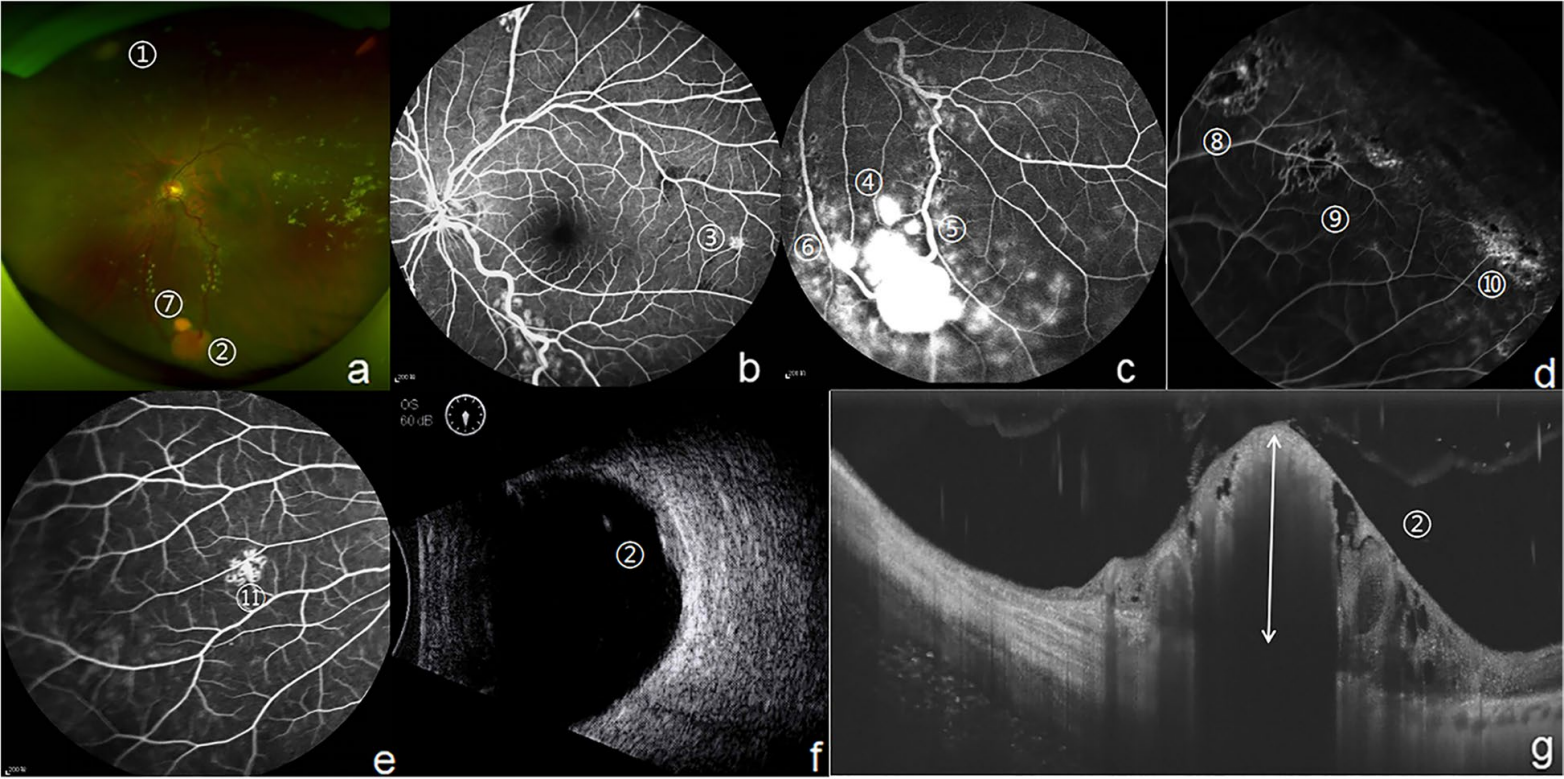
The first multimodal image evaluation of eleven RCHs in a Von Hippel-lindau syndrome patient on June 6^th^, 2017. a. Fundus photo identified huge RCHs (No.1, 2, 7 RCHs). b. FFA visualized a newly developed small RCH in temporal retina (No.3 RCH). c-e. FFA sketched the contours of several small peripheral RCHs (No.4–11 RCHs). f. B-scan ultrasonography displayed the largest oblate lesion with the size of 6 mm* 6 mm*2 mm was located in the inferior nasal retina (No.2 RCH). F. SS-OCT (single line scan mode) showed an elevated lesion wrapped much exudation and the largest RCH with the size of 2.15*2.60*1.35 mm, arrow represent the height (1.35 mm). (No.2 RCH). *RCH* retinal capillary hemangioblastomas, *FFA* fluorescein fundus angiography, *SS-OCT* swept-source optical coherence tomography
